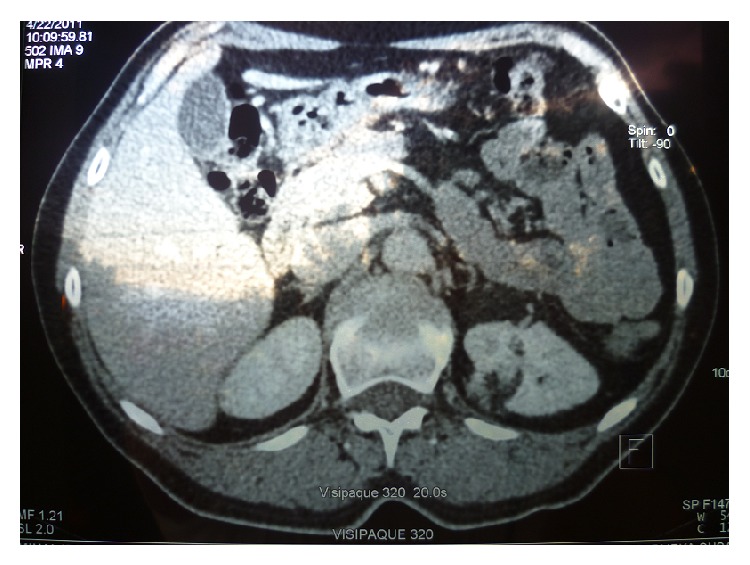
Six months after the spontaneous rupture of the angiomyolipoma (AML) on the left kidney, control CT showed the complete regression of the retroperitoneal hematoma and a well-delineated AML.
